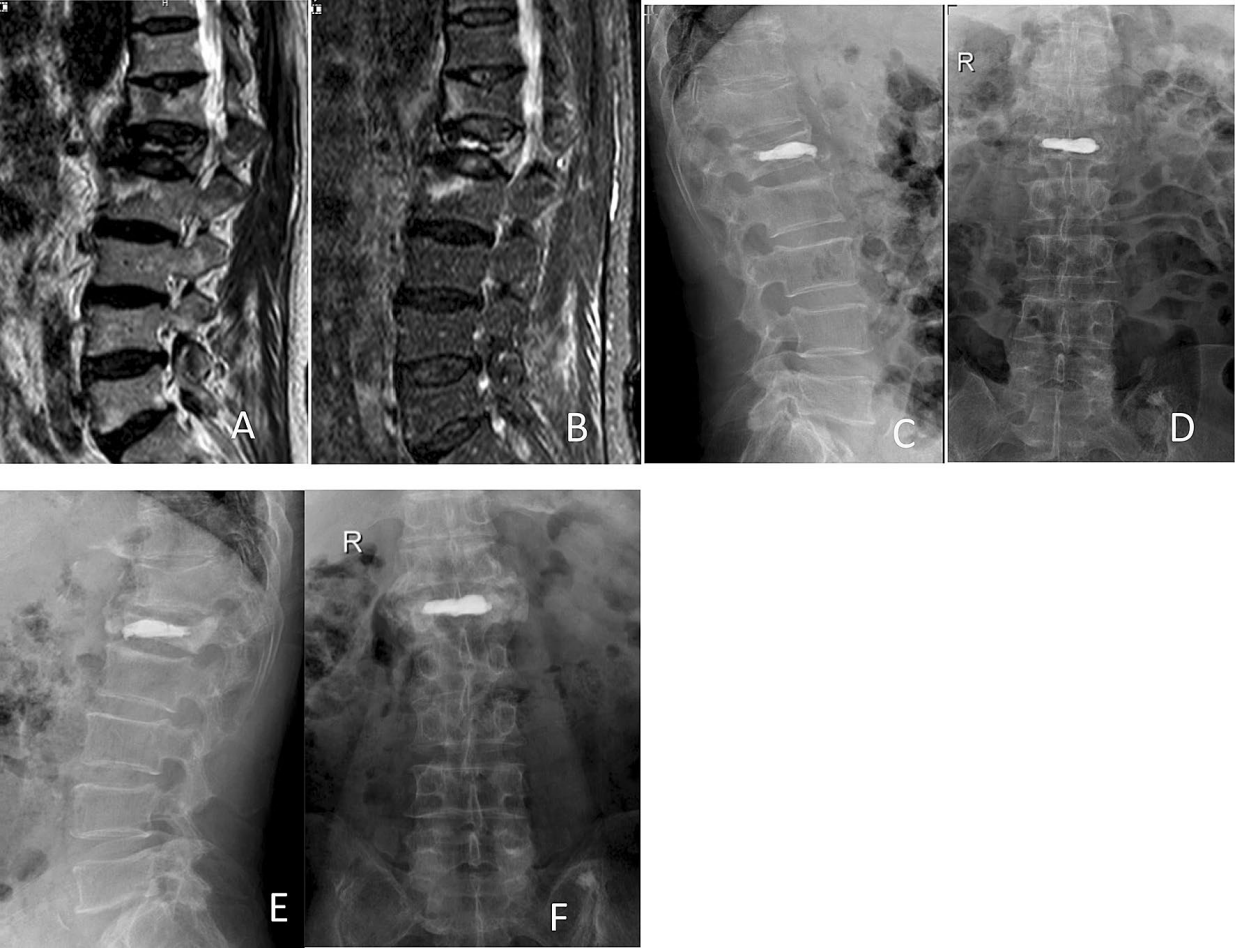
Preoperative and postoperative films of a 65-year-old woman with OVCFs of L1 vertebral body, treated with high-viscosity cement PVP. Preoperative sagittal MR image showing significant loss of the anterior vertebral body height at the L1 level (A), (B). Anterior–posterior and lateral films showing no leakage of bone cement postoperatively (C), (D) and at 1 year postoperatively (E), (F).
